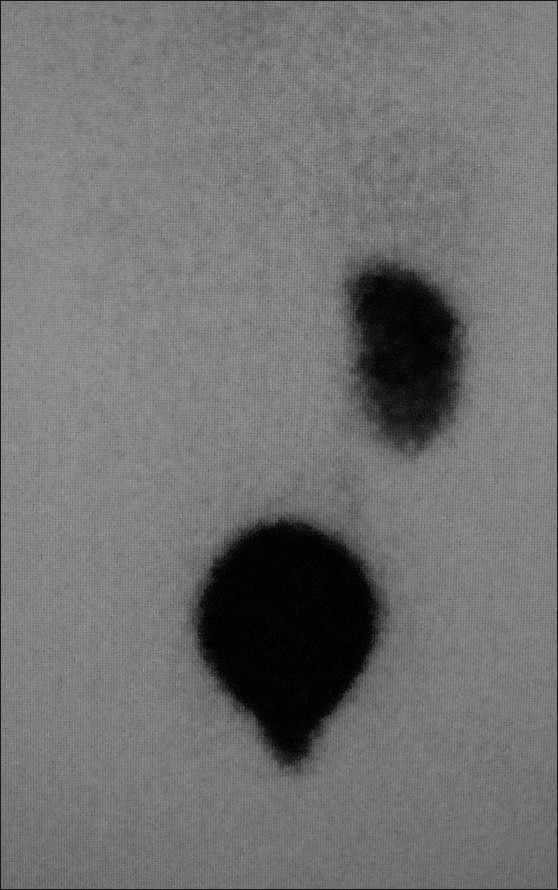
Posterior image from a Tc99m DMSA scintigraphy study reveals absence of the left kidney
